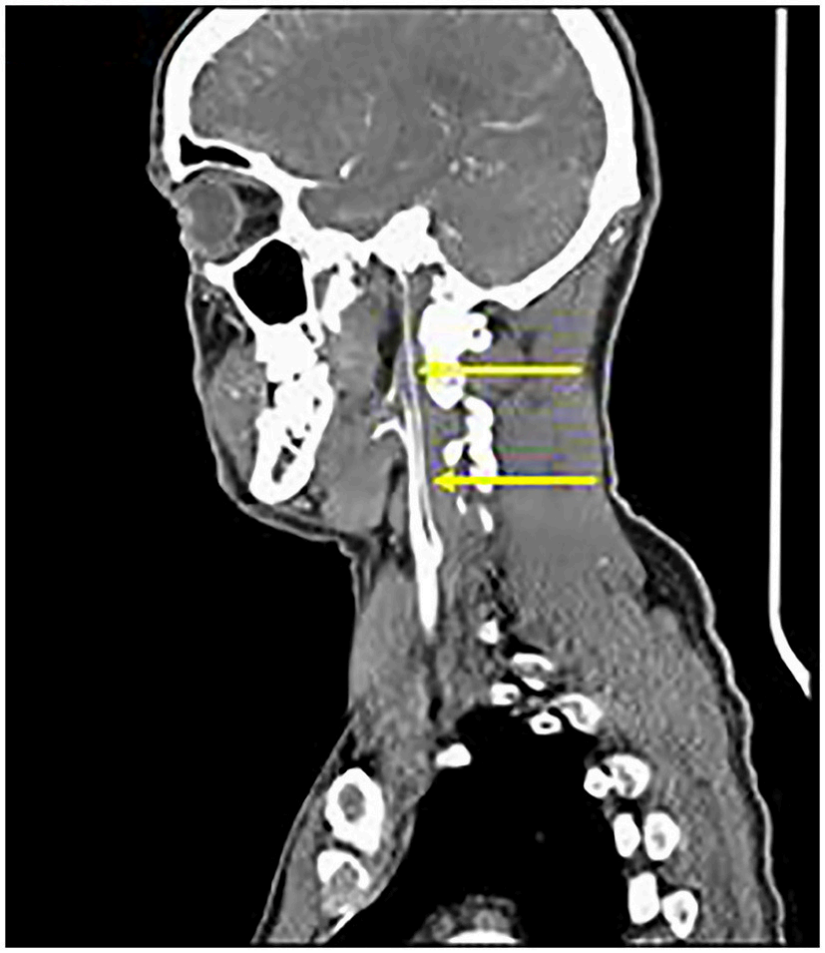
Extracranial CTA: Diffuse severe left ICA narrowing from 0.5 cm beyond the carotid bifurcation (arrows), consistent with “Alagille vasculopathy.”
